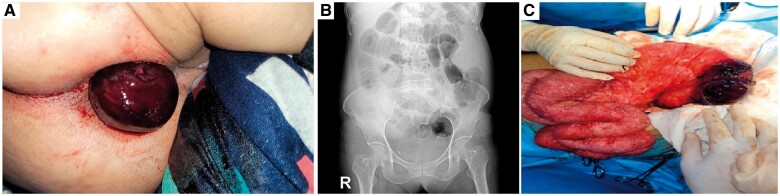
The clinical data of the case with ileorectal intussusception. (A) The anal mass. (B) Abdominal X-ray imaging showing the obstruction. (C) Reduction of the protruding anal mass. Necrosis of the protruding mass was seen.
